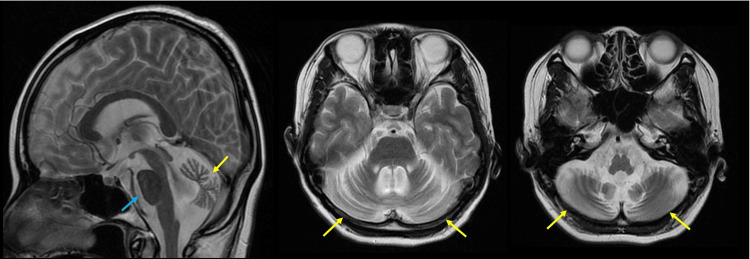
The result of brain magnetic resonance imaging (MRI) at 32 years old. T2-weighted brain MRI shows significant cerebellar atrophy (yellow arrows) and mild brainstem atrophy (blue arrow), with minimal changes compared to the results at 28 years old.
